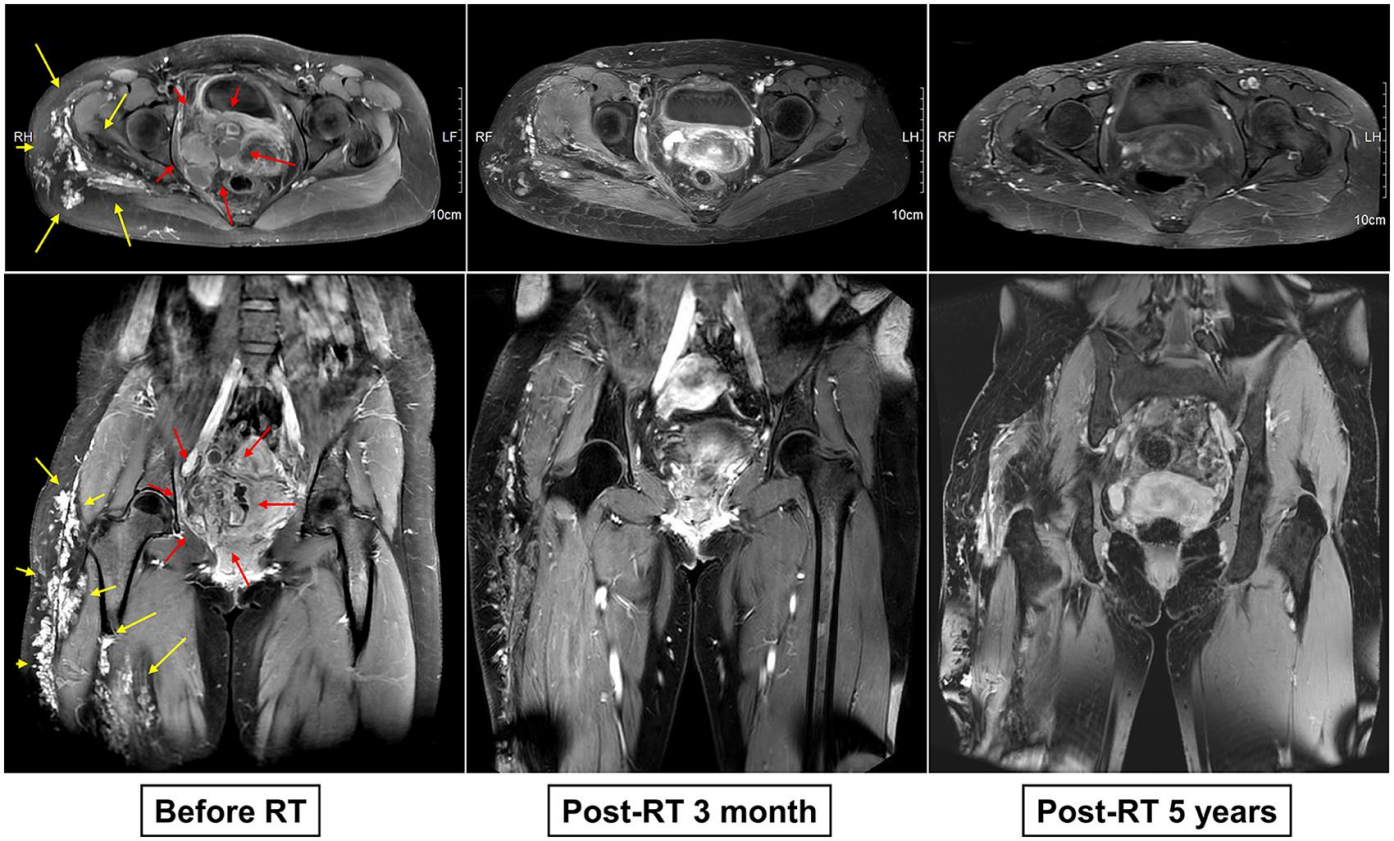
Serial axial and coronal pelvic MRI images before and after radiotherapy. Axial and Coronal MRI scans were obtained at regular intervals before and after radiotherapy (RT), demonstrating a gradual reduction in the vascular lesion size.
